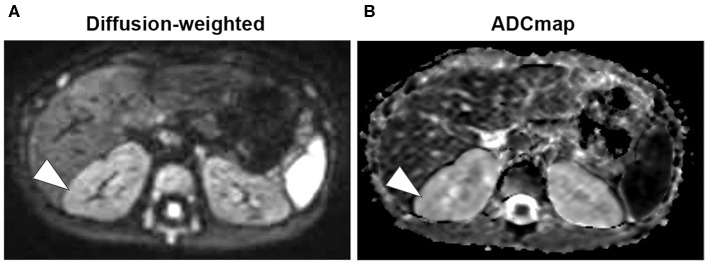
Magnetic resonance imaging (Day 428). (A) Diffusion-weighted images showed that the mass with high-signal areas of thick-walled structures disappeared (arrowhead). (B) Low-signal masses are also not visible on apparent diffusion coefficient (ADC) map (arrowhead).
